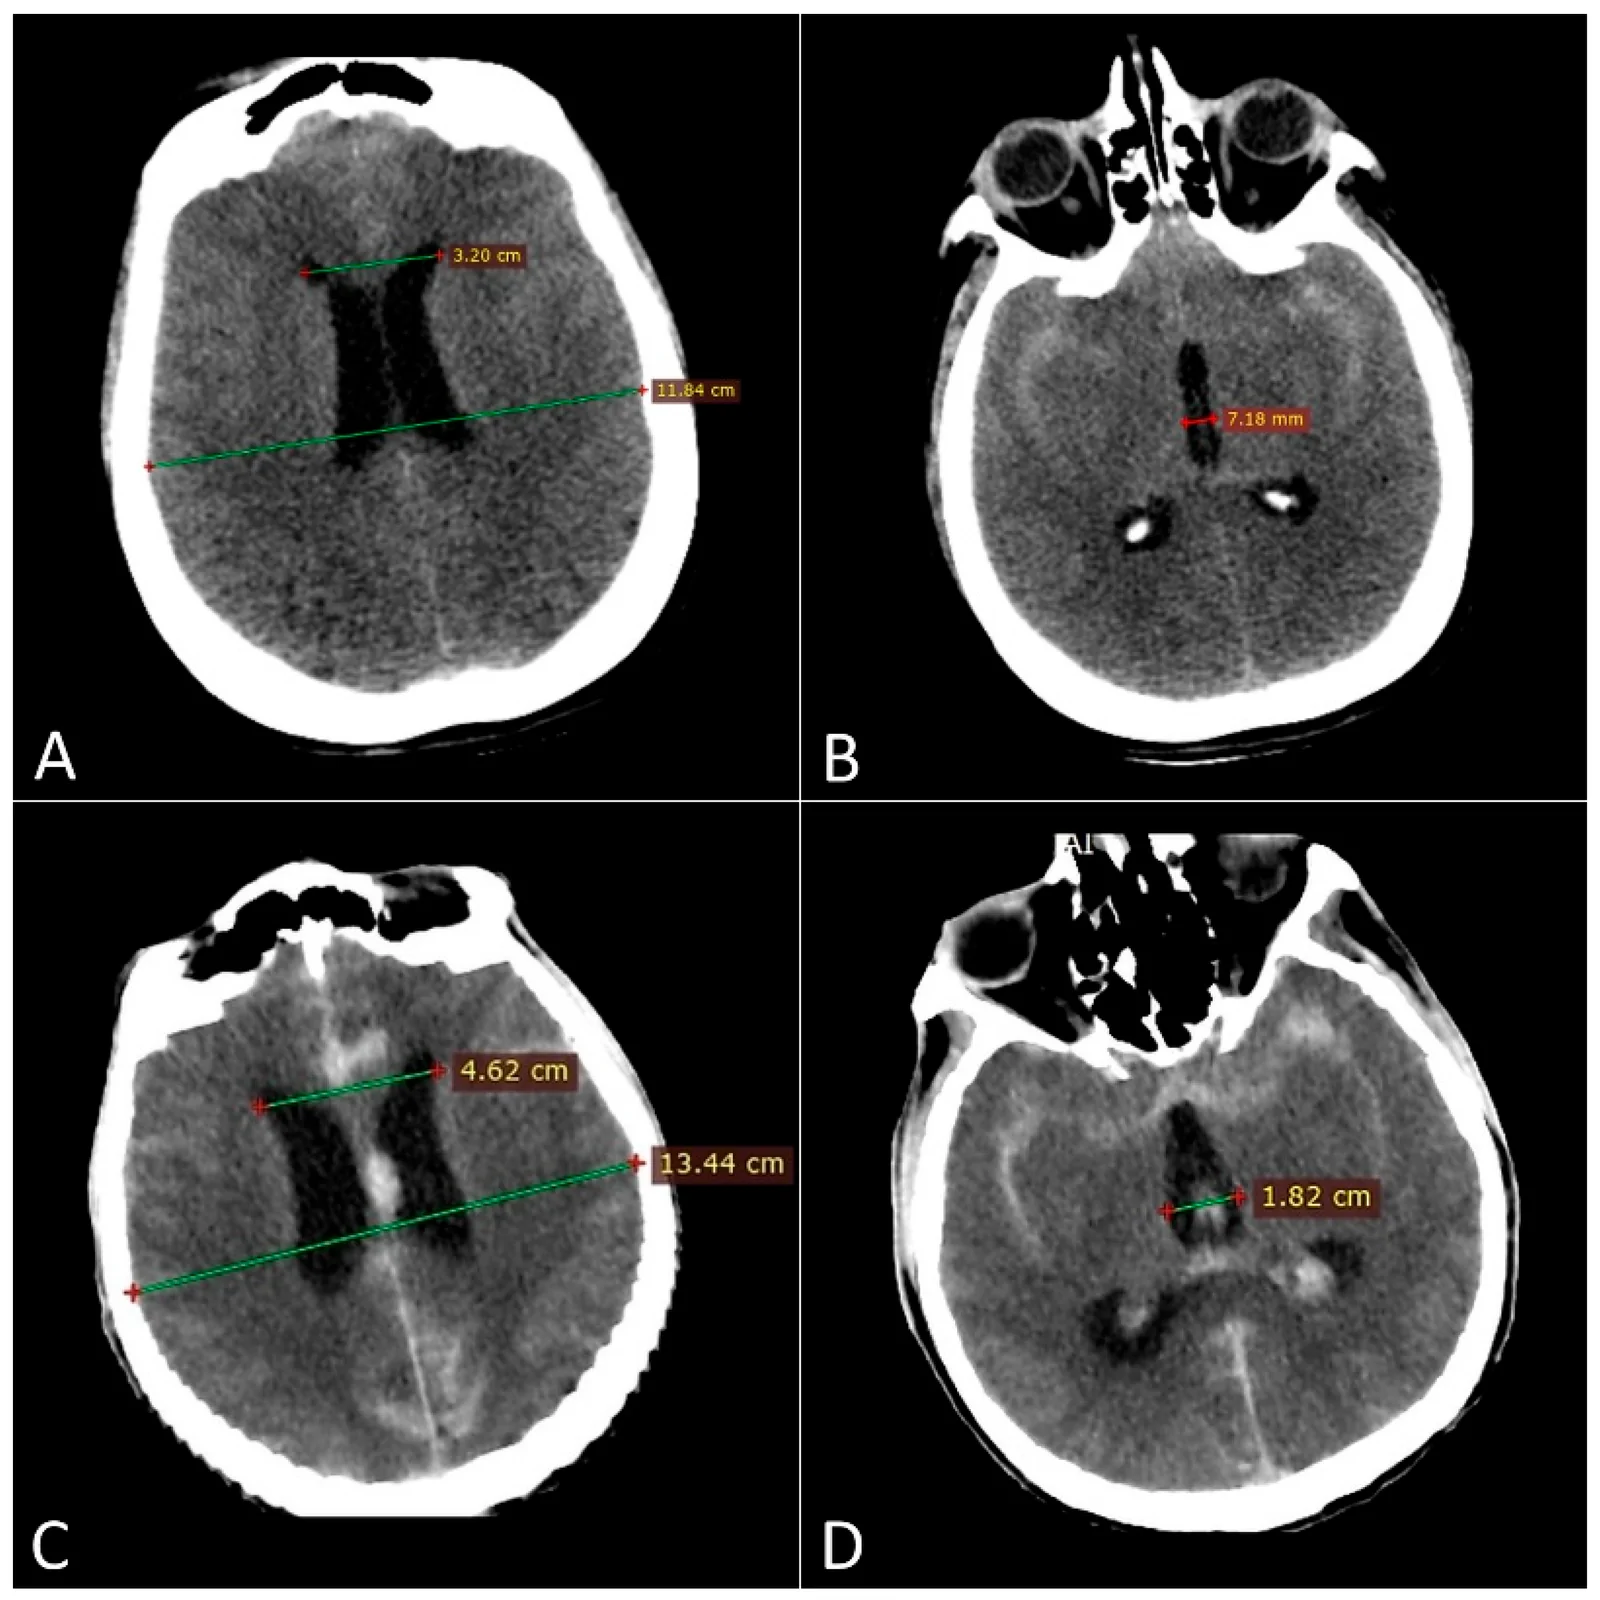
Representative axial cranial CT images demonstrating ventricular measurements in patients with low- and high-risk profiles for shunt-dependent hydrocephalus following aneurysmal subarachnoid hemorrhage. (A) Evans index measurement in a low-risk patient. (B) Third ventricular width measurement in a low-risk patient. (C) Evans index measurement in a high-risk patient with more prominent ventricular dilatation. (D) Third ventricular width measurement in a high-risk patient.
